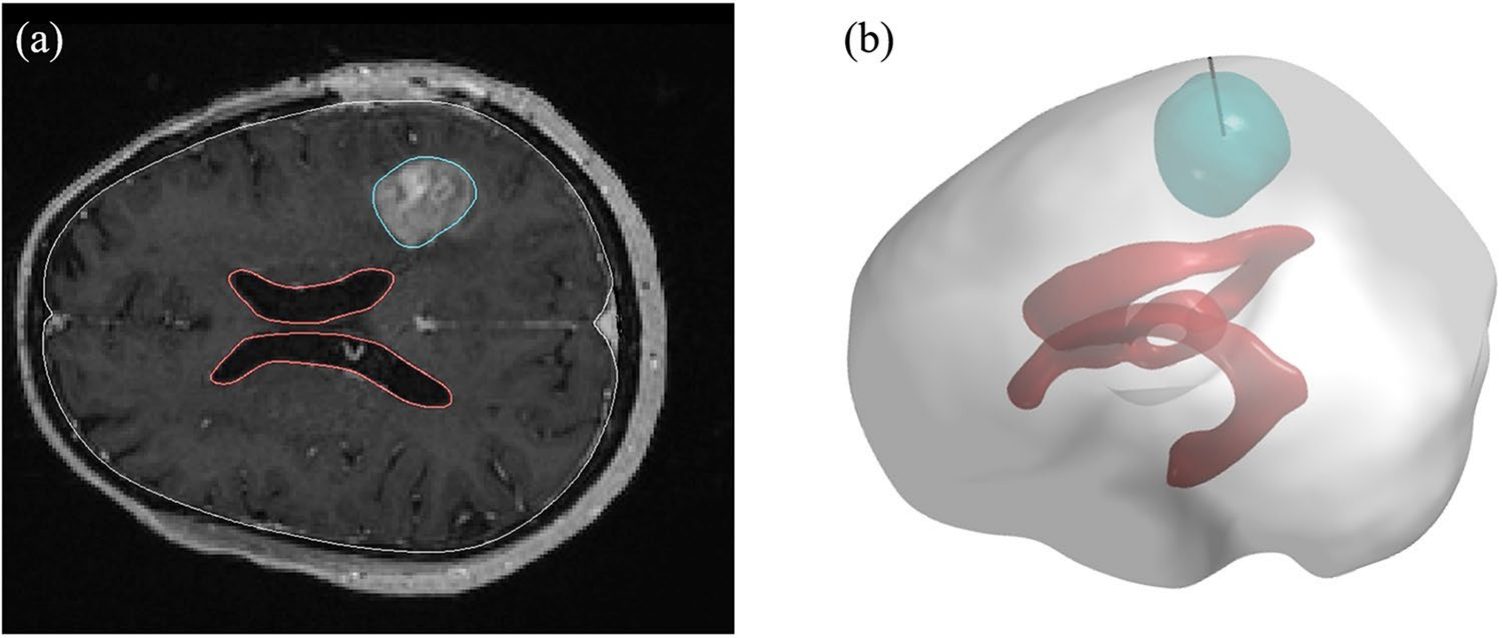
Model geometry. (a) The representative slice of MR images used for geometry reconstruction, (b) the reconstructed 3-D geometry
